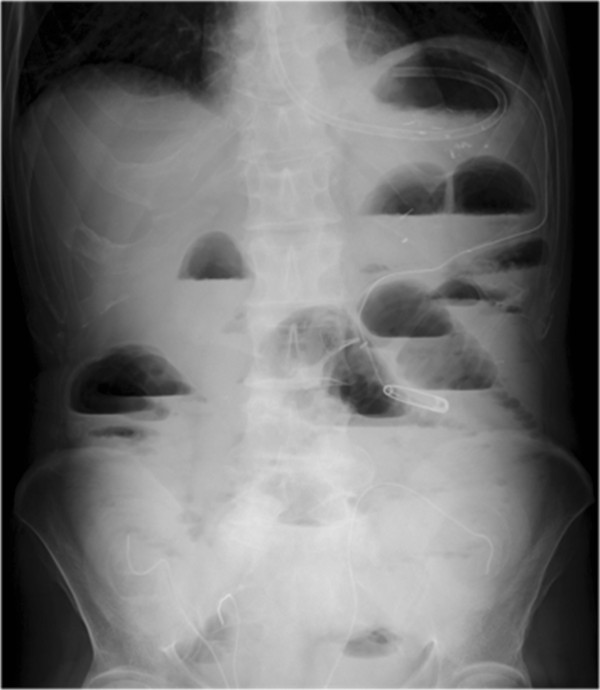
Plain standing abdominal X-ray. Niveau formation in the small intestine.
